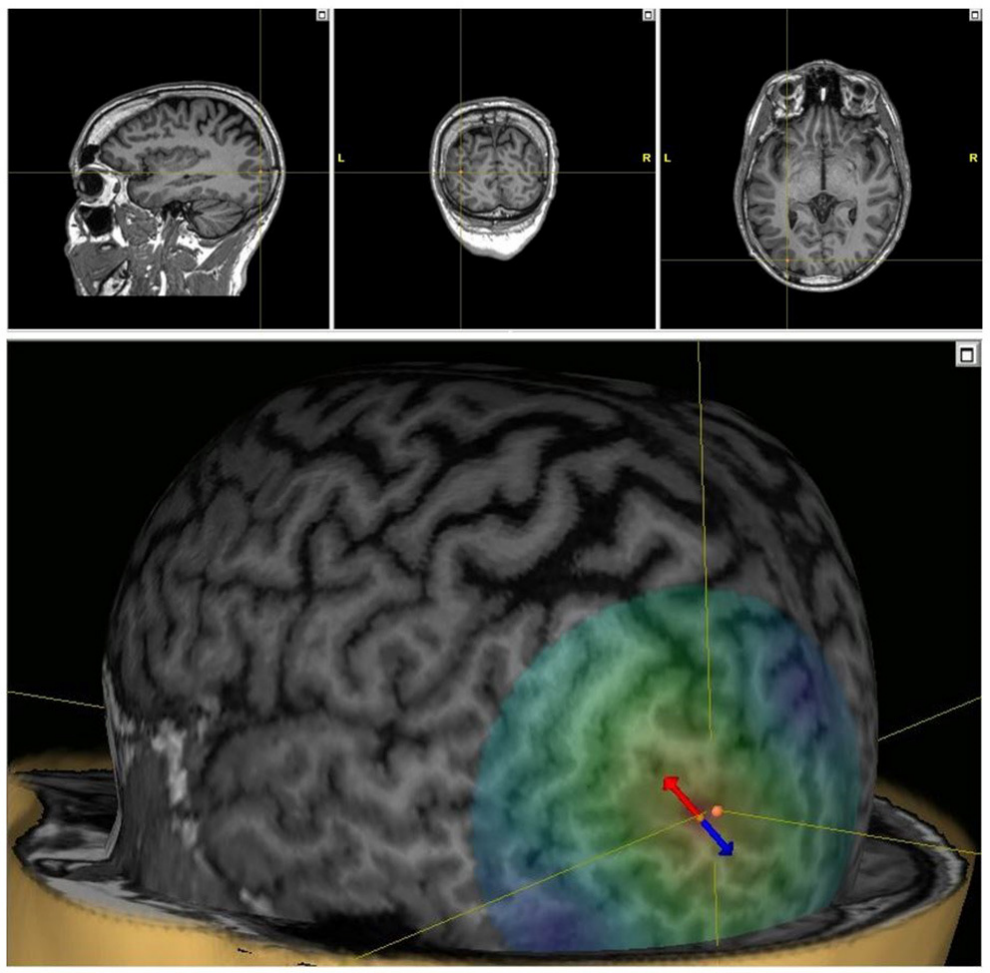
TMS targeting. Stereotactic TMS targeting using the patient's MRI in 3 planes (top) and 3D model (bottom). The dipole of the TMS pulses is indicated, with the arrows indicating the direction of the induced electric field (red cathodal, blue anodal).
